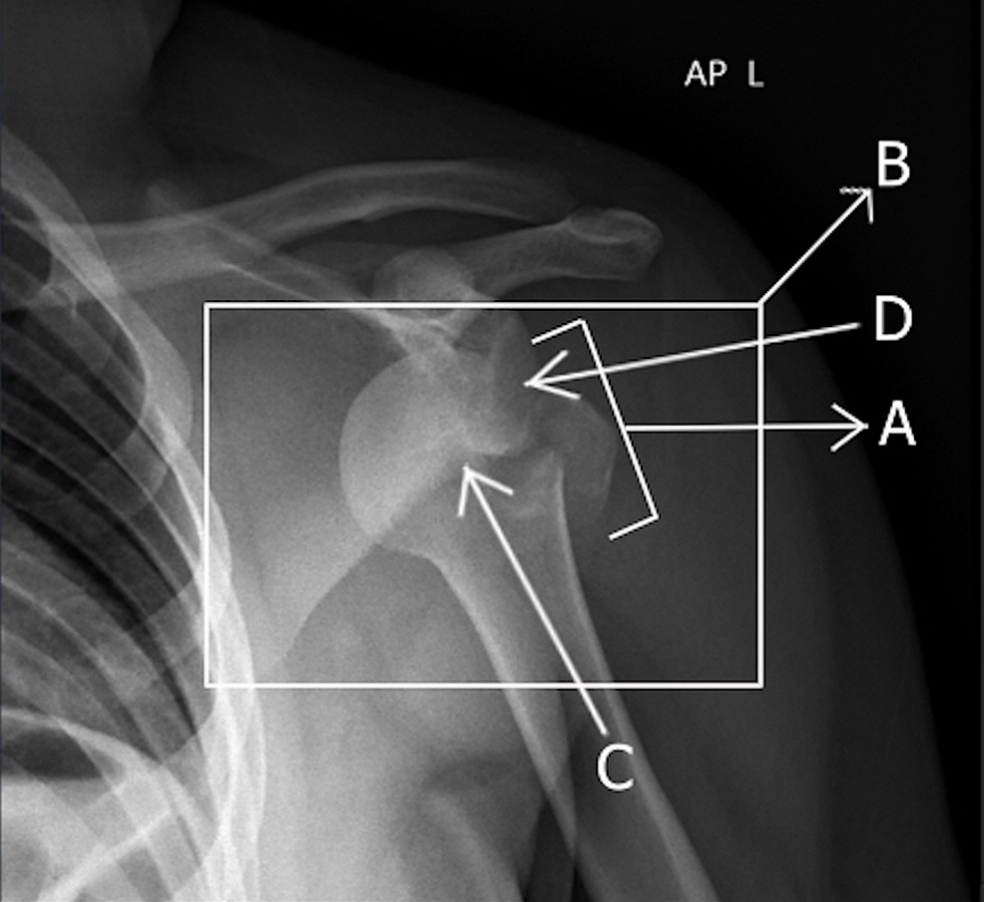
Anterior-posterior X-ray showing the anterior left glenohumeral joint dislocation with an avulsion fracture of the greater tuberosity. The avulsion fracture of the greater tuberosity (A), and the anterior left glenohumeral joint dislocation (B) can be seen. The humeral head (C) is dislocated in an anterior direction to the glenoid (D).
